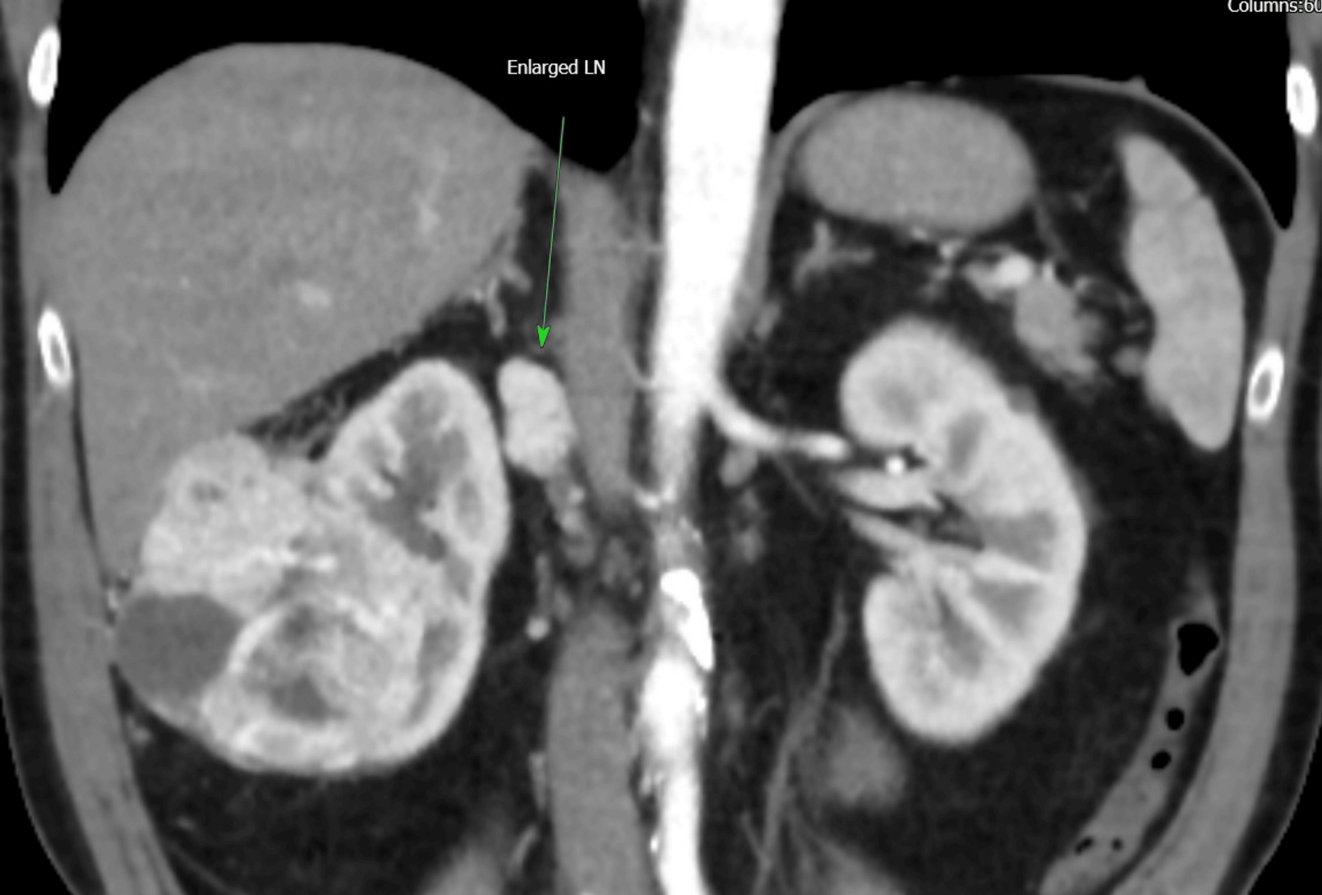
Coronal section of CT scan showing enlarged hilar lymph nodes CT: computed tomography
